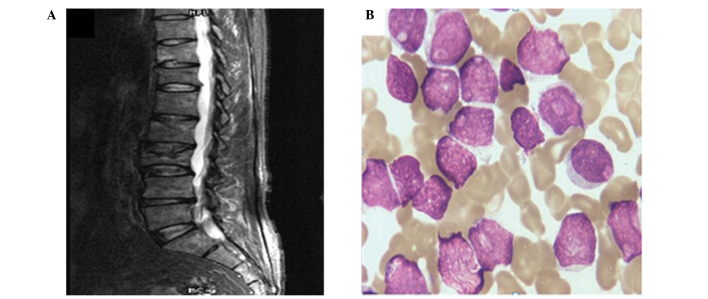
(A) Magnetic resonance imaging revealed impaired physiological curve of thoracolumbar spine, clusters of iso/hypo mixed signals and pathological fracture in T8 and L3. (B) Bone marrow aspiration demonstrated active hyperplasia and a blast cell count of 90.0%.
